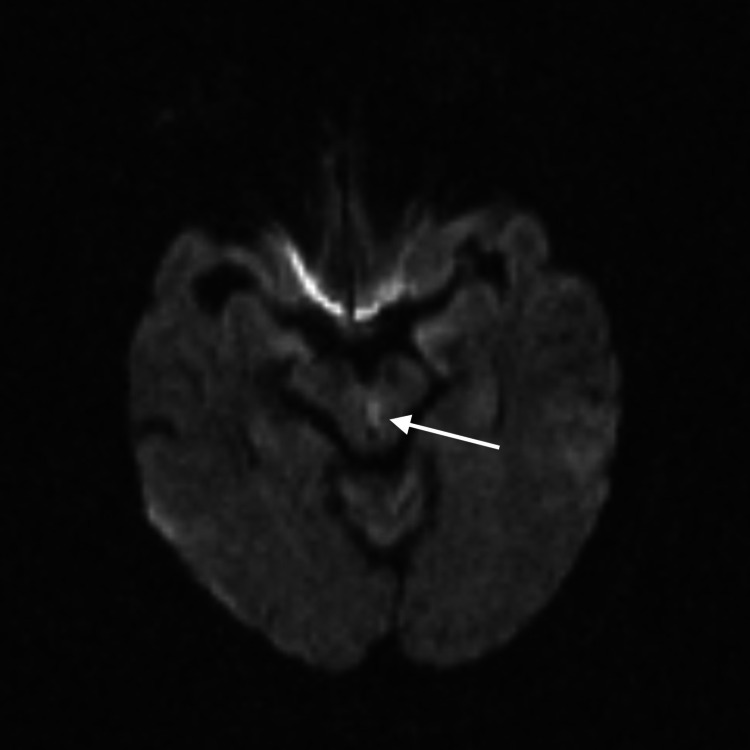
Brain MRI showing a linear area of restricted diffusion within the midbrain tegmentum. MRI: magnetic resonance imaging
